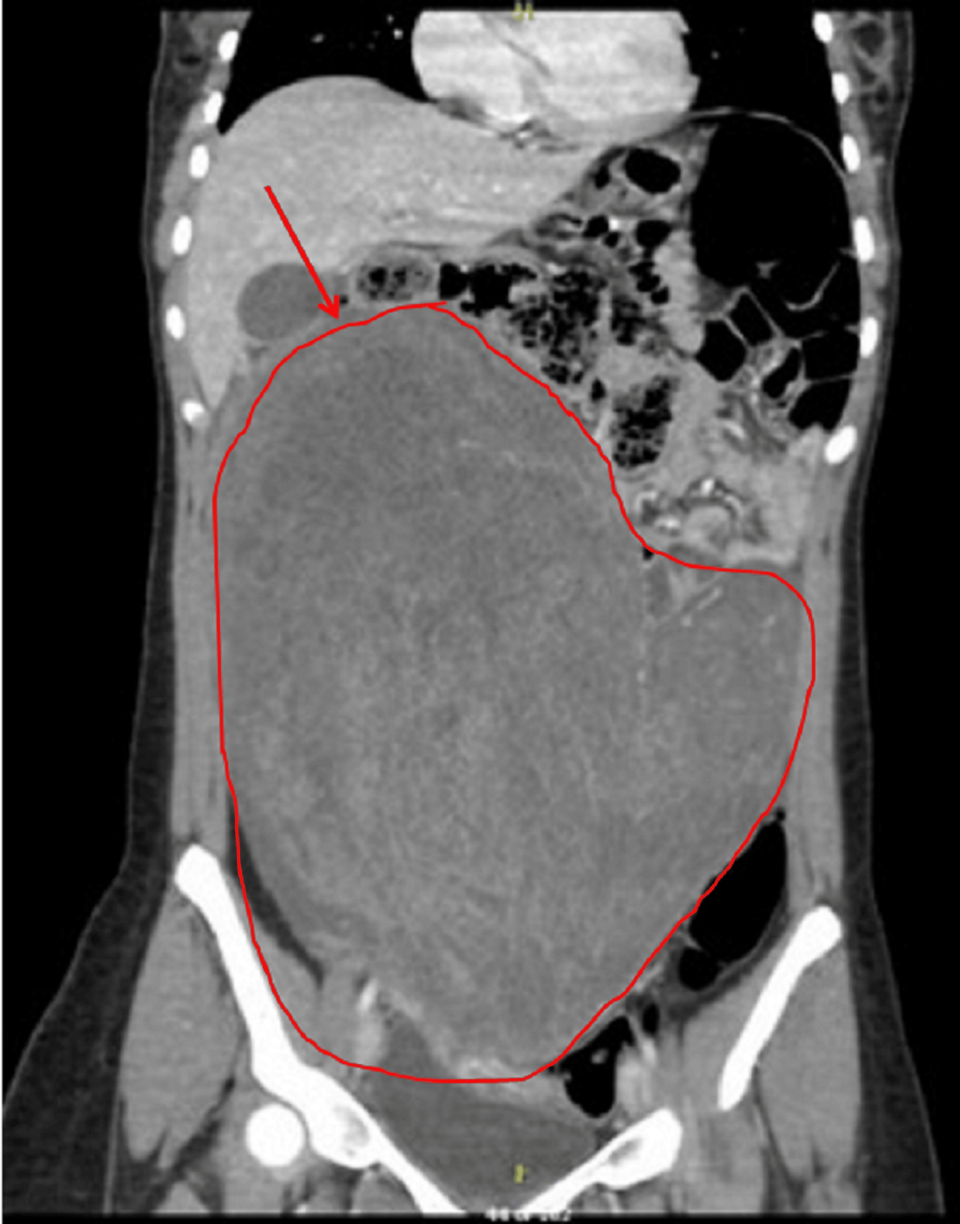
Coronal CT scan showing the mass compressing bladder and bowel (Case 2)
